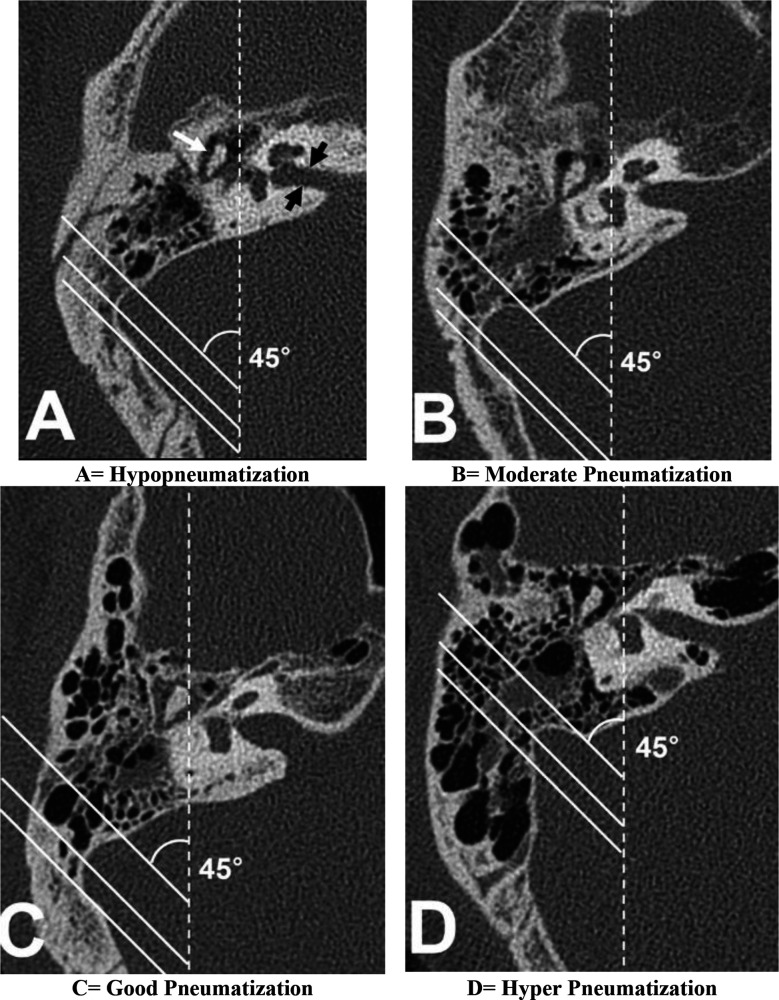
HRCT of patients of the current study showing types of pneumatization of Temporal bone including the mastoid process
